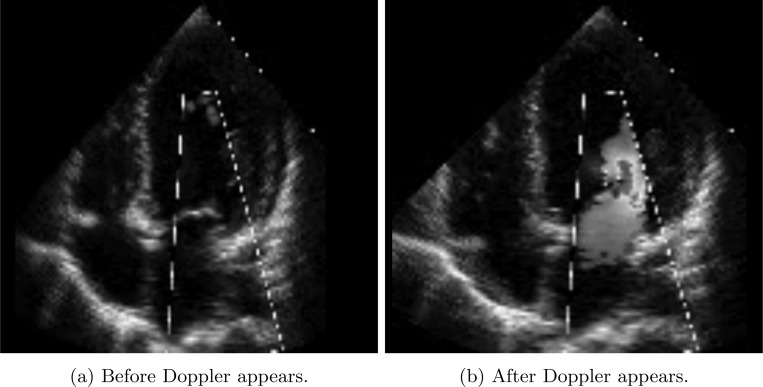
Example of TTE with Doppler.
